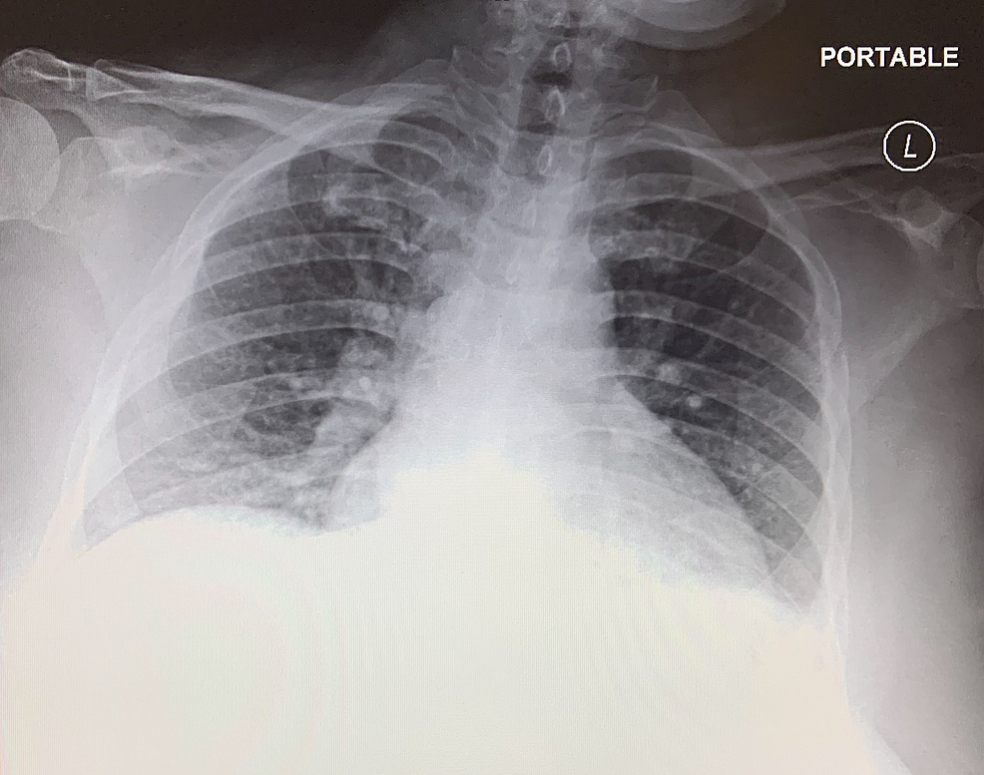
X-ray demonstrating decreased lung volumes with central bronchovascular crowding and bibasilar sub-segmental atelectasis in addition to several indeterminate lytic lesions of the ribs bilaterally.
